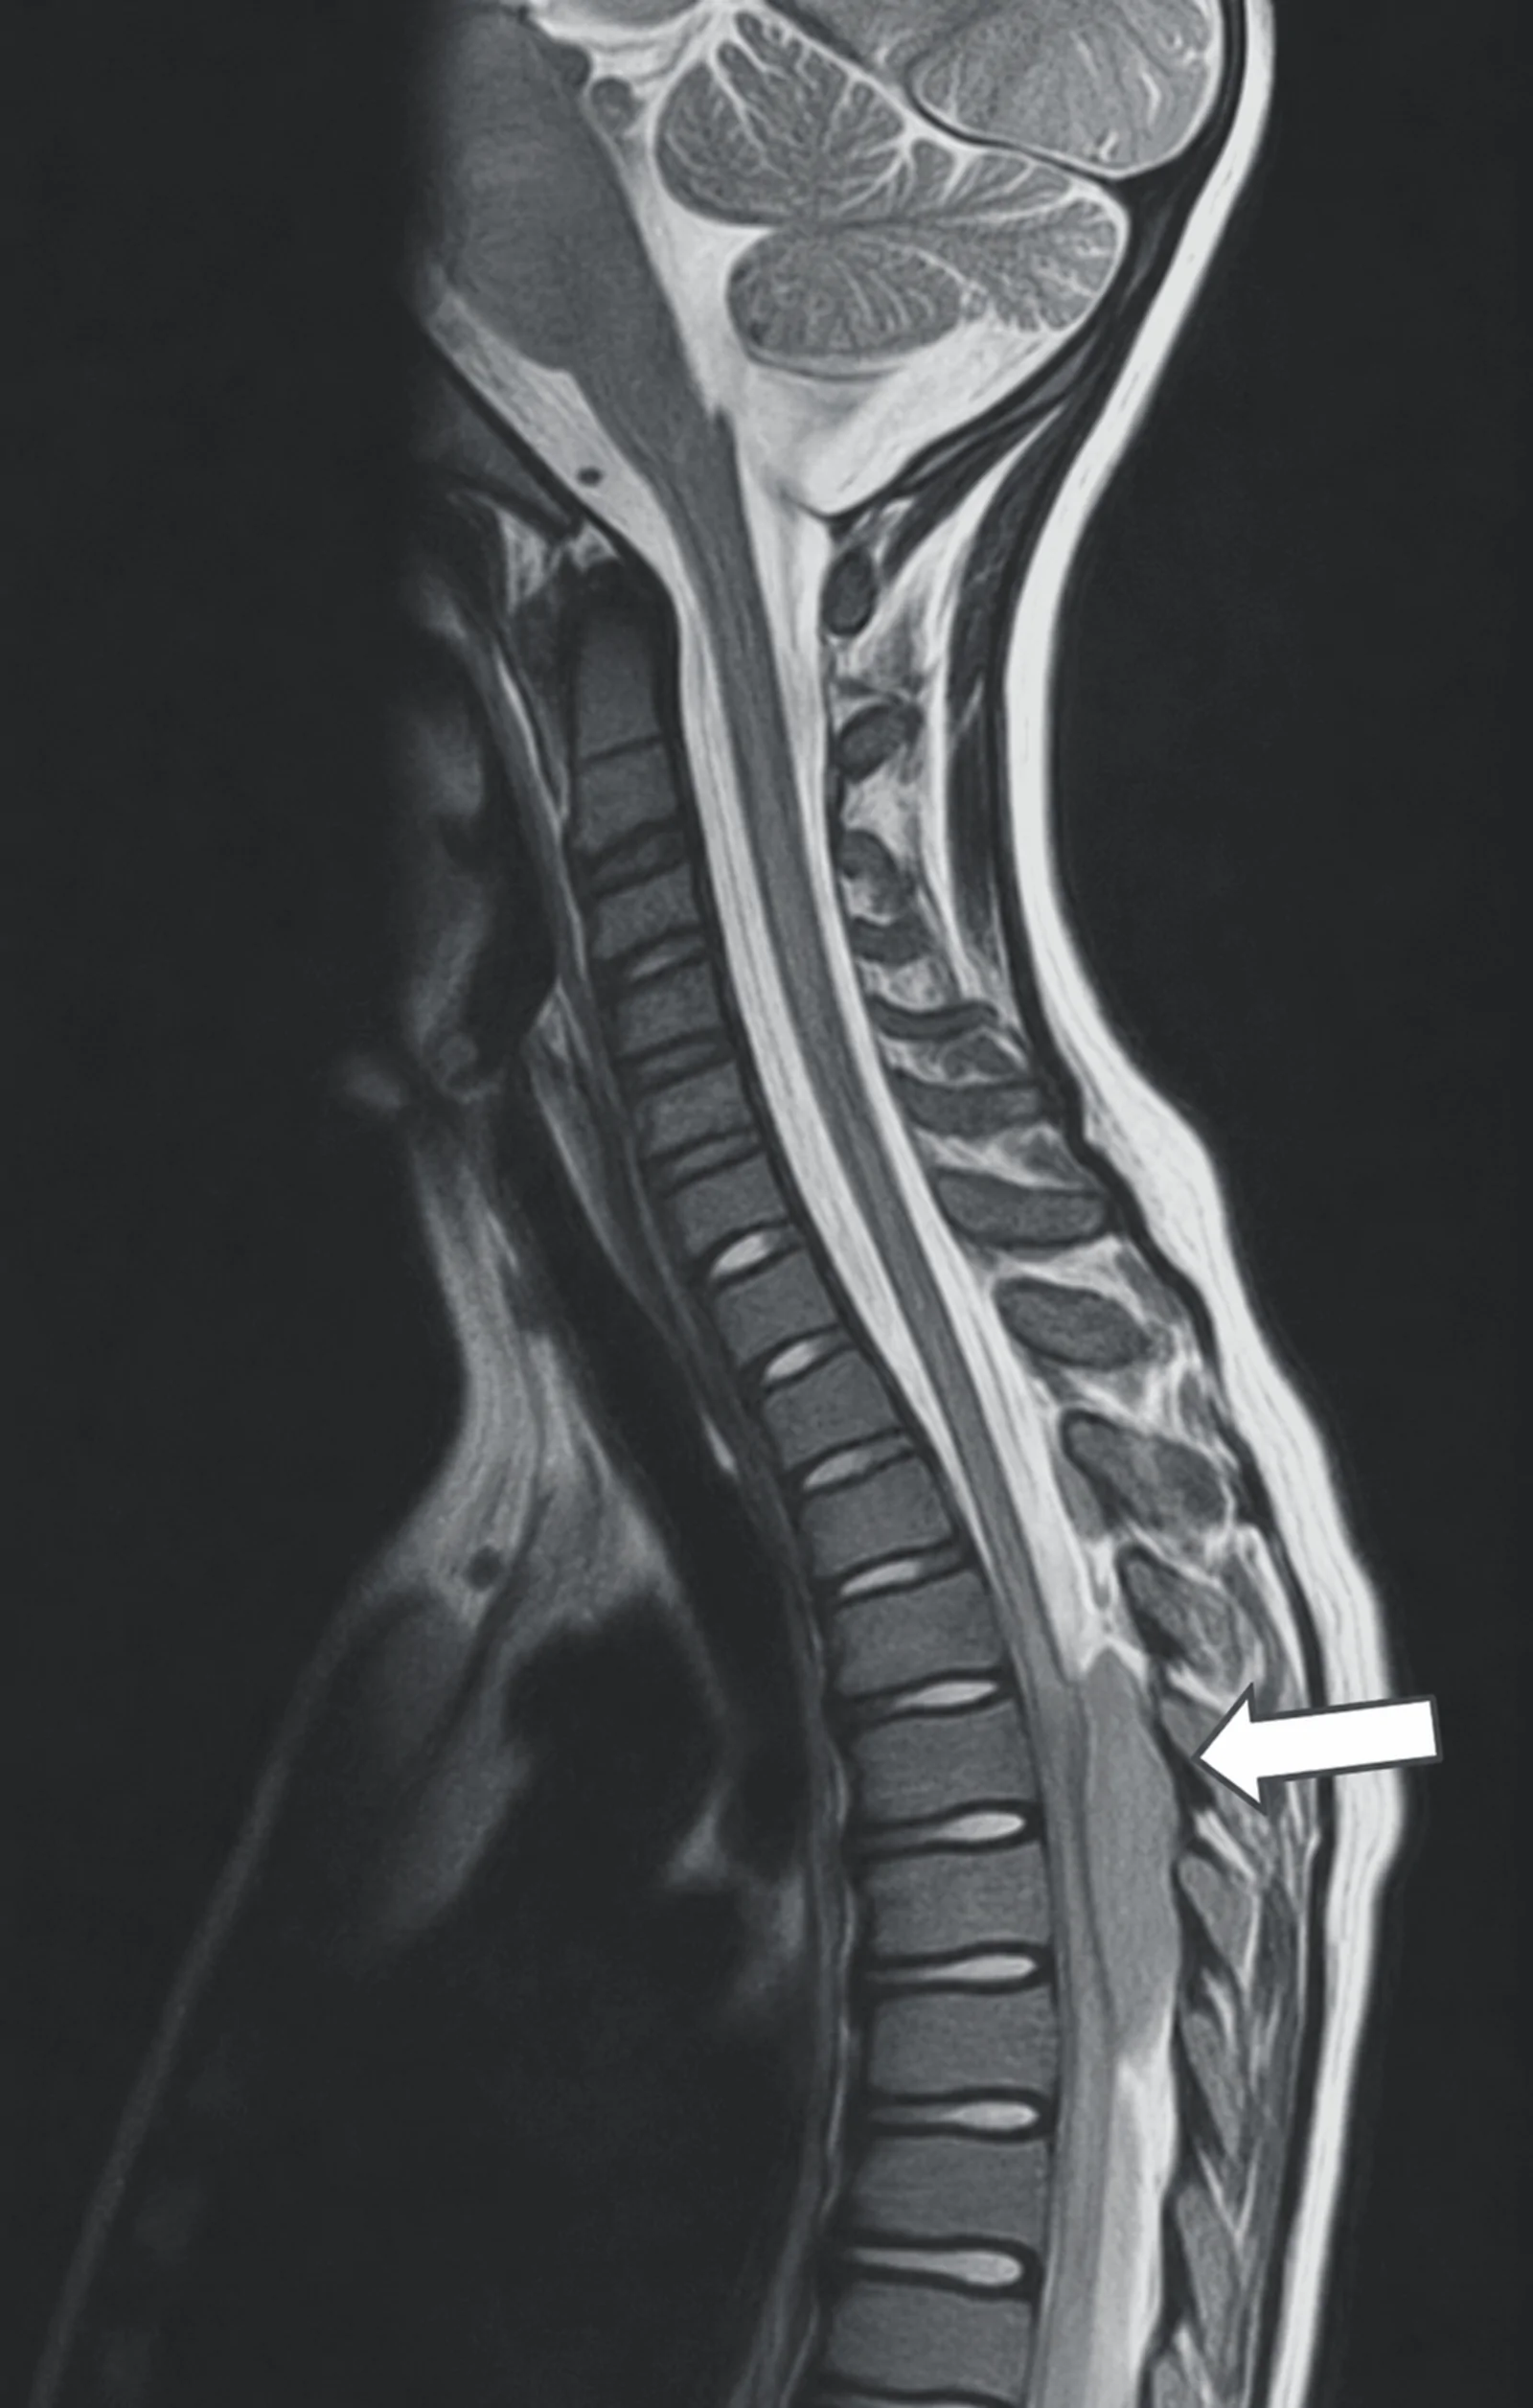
Preoperative sagittal T2-weighted spinal MRI showing a well-defined posterior epidural mass measuring 12 × 9 × 45 mm and extending from D4 to D6 (white arrow). The lesion appears hyperintense on T2-weighted images and is causing severe spinal cord compression with associated intramedullary T2 hyperintensity, consistent with secondary spinal cord edema due to mass effect. It also extends into the bilateral D4–D5 and D5–D6 neural foramina and is in close contact with the posterior vertebral arches, without evidence of vertebral body involvement.
